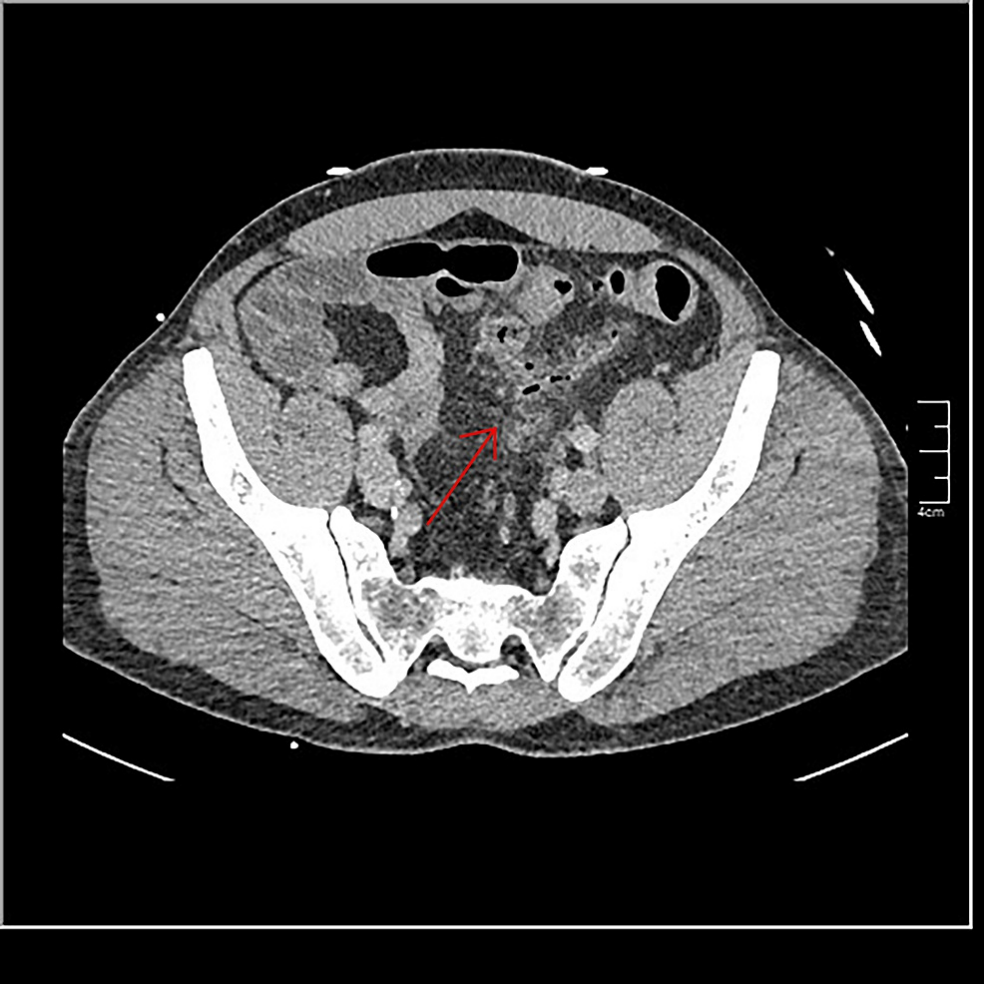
Abdominal CT scan with an injection of contrast showing thickening of the sigmoid colon with infiltration of the surrounding fat. CT, computed tomography
